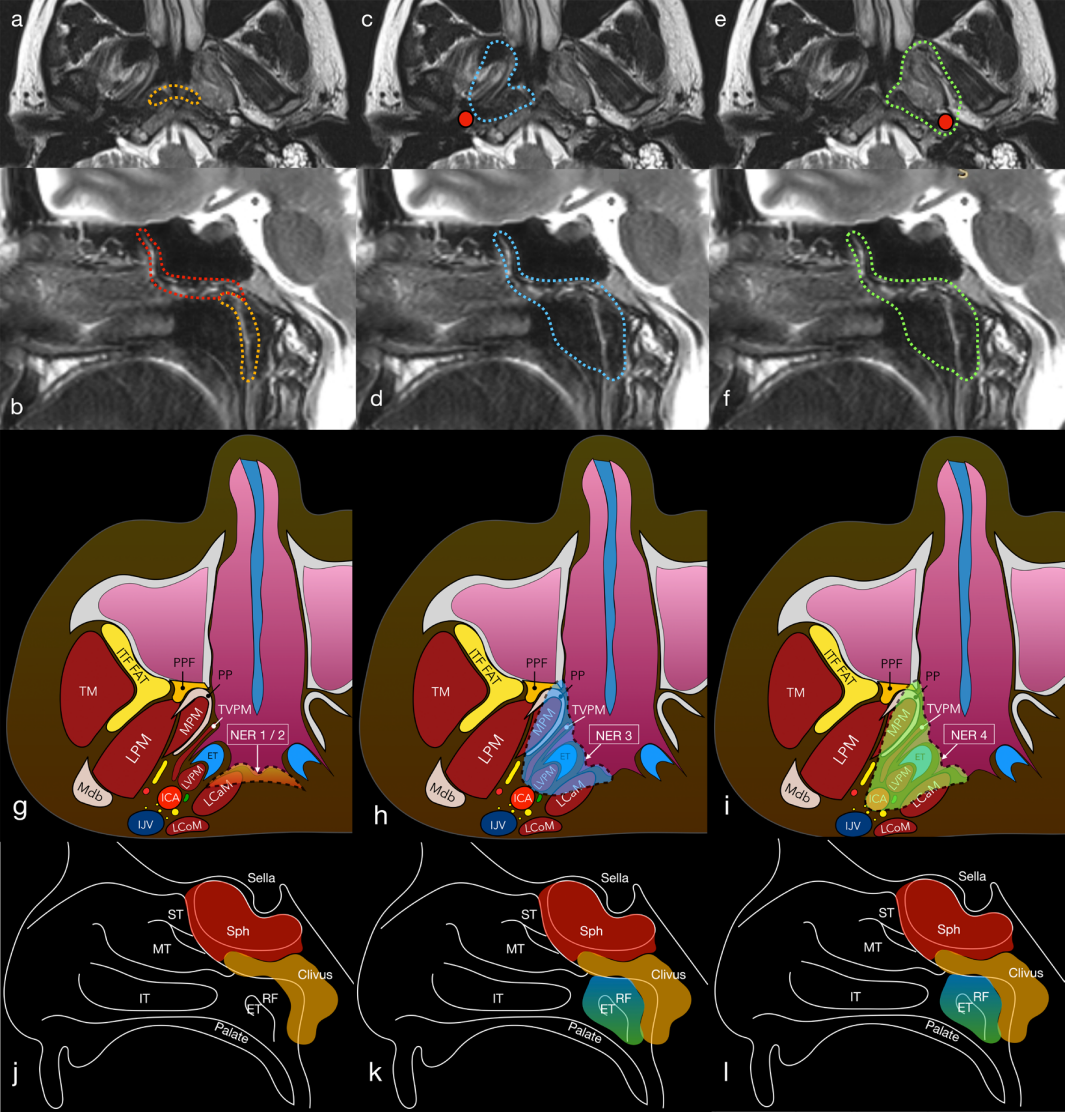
Surgical resection limits of the different types of NERs are highlighted by the dotted lines on magnetic resonance images of a patient with a left‐sided nasopharyngeal malignancy abutting the ipsilateral internal carotid artery (a–f). NER type 1 and 2 surgical limits seen on an axial plane (a), corresponding laterally to the Rosenmüller fossa (lateral recess of the nasopharynx), preserving the Eustachian tube; NER type 1 (yellow) and 2 (yellow + red) surgical limits seen on a sagittal plane (b), corresponding inferiorly to the naso‐oropharyngeal passage and superiorly to the floor of the sphenoid sinus, in case of NER 1, or including the floor and anterior wall of the sphenoid sinuses in case of NER 2; NER type 3 surgical limits seen on axial (c) and sagittal (d) planes, corresponding superiorly and inferiorly to those of the NER type 2 but more laterally extended to include the Eustachian tube; the ICA is highlighted with a red circle; type 4 NER seen on axial (e) and sagittal (f) planes. The ICA, highlighted with a red circle, is included in the resection which is further laterally extended compared with NER type 3. The surgical limits relative to relevant anatomical structures can be seen in images g to l, with yellow and red areas corresponding to NER 1 and 2 respectively, the blue area corresponding to NER type 3, and the green area to NER type 4. ET, Eustachian tube; ICA, internal carotid artery; IJV, internal jugular vein; IT, inferior turbinate; ITF, infratemporal fossa; LCaM, longus capitis muscle; LCoM, longus colli muscle; LPM, lateral pterygoid muscle; LVPM, levator veli palatini muscle; Mdb, mandible; MPM, medial pterygoid muscle; MT, middle turbinate; PP, pterygoid process; PPF, pterygopalatine fossa; RF, Rosenmüller fossa; Sph, sphenoid sinus; ST, superior turbinate; TM, temporalis muscle; TVPM, tensor veli palatini muscle. [Color figure can be viewed at wileyonlinelibrary.com]
